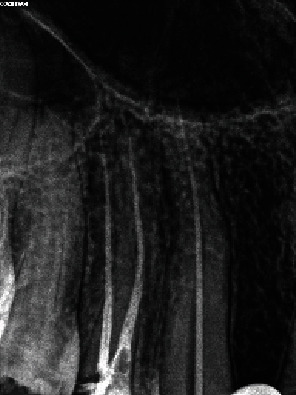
Periapical radiograph confirming master gutta-percha reaching the full working length.
